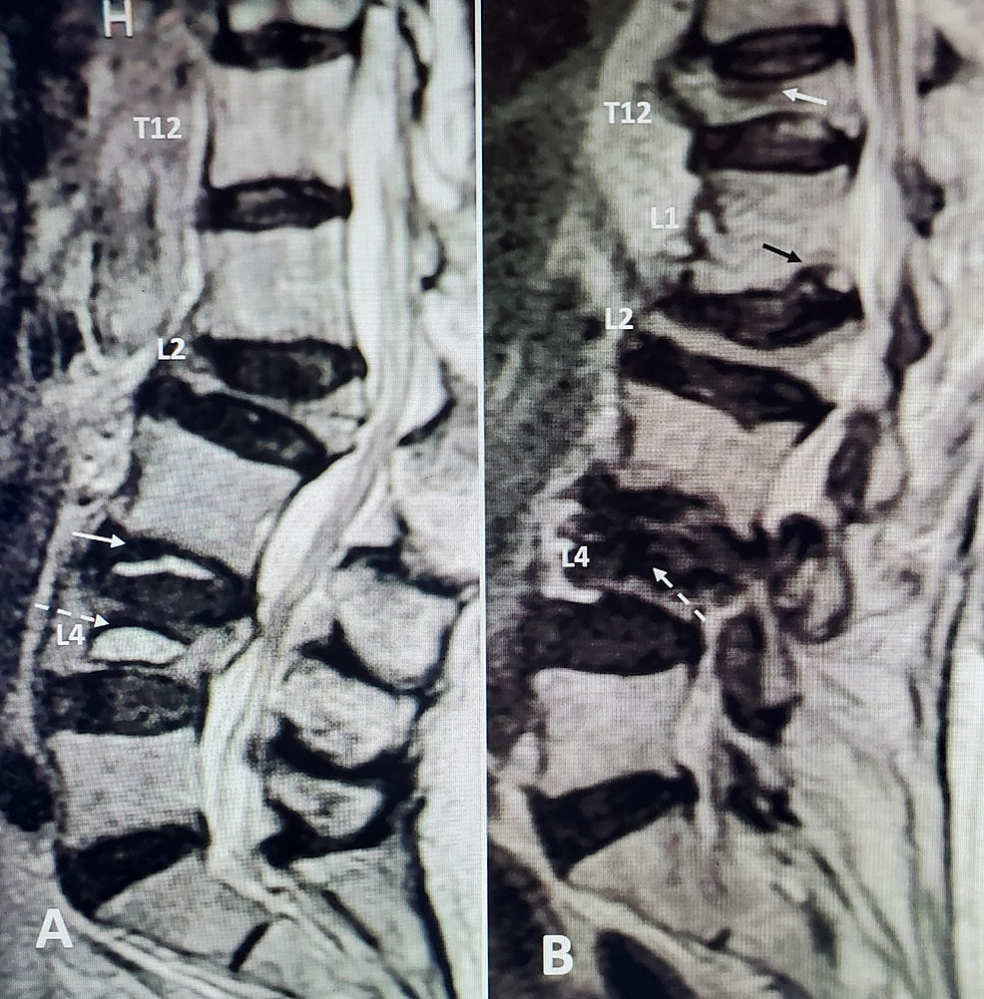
Multiple high-degree and vertebra plana fractures seen over a 24-month period of time A: Initial MRI when patient only had lumbar pain due to L4 high-degree fracture with vertebral clefts (solid white arrow) indicative of both acute fracture and micro-instability. Although the L2 vertebra plana was present three years previously, there was no pain at that level and a bone scan did not show uptake at L2 but only at L4. The L4 fracture was treated with balloon kyphoplasty. B: The same patient re-evaluated for thoracic pain 18 months later. There is a new T12 70% "pancake" fracture with almost complete collapse under superior endplate of T12 (solid white arrow). There is a small inferior endplate fracture posteriorly at L1 (solid black arrow). The old L2 fracture is unchanged. The L4 fracture with black signal bone cement is partially re-expanded (dashed white arrow).
